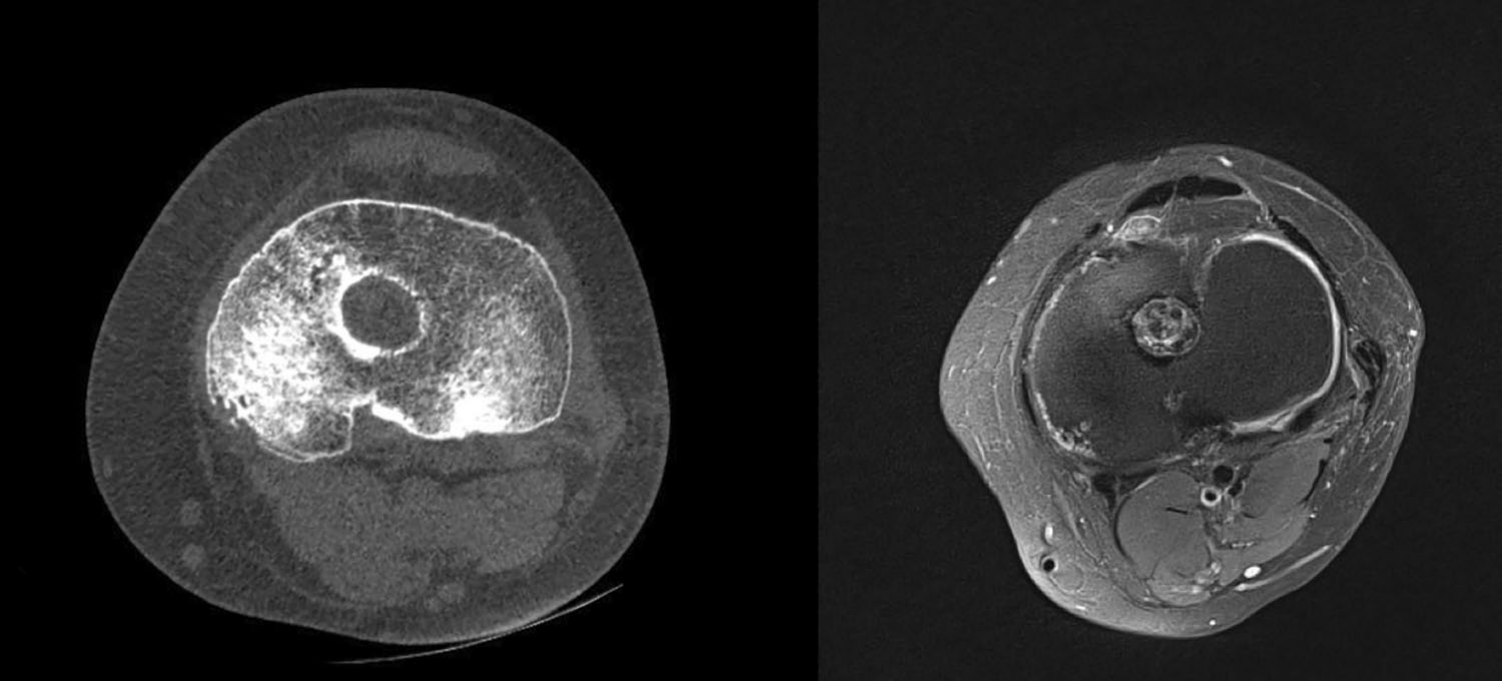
Pre-operative axial CT-Scan (on the left) and MRI (on the right) of a re-revision surgery case showing an enlargement of the previous tibial tunnel
